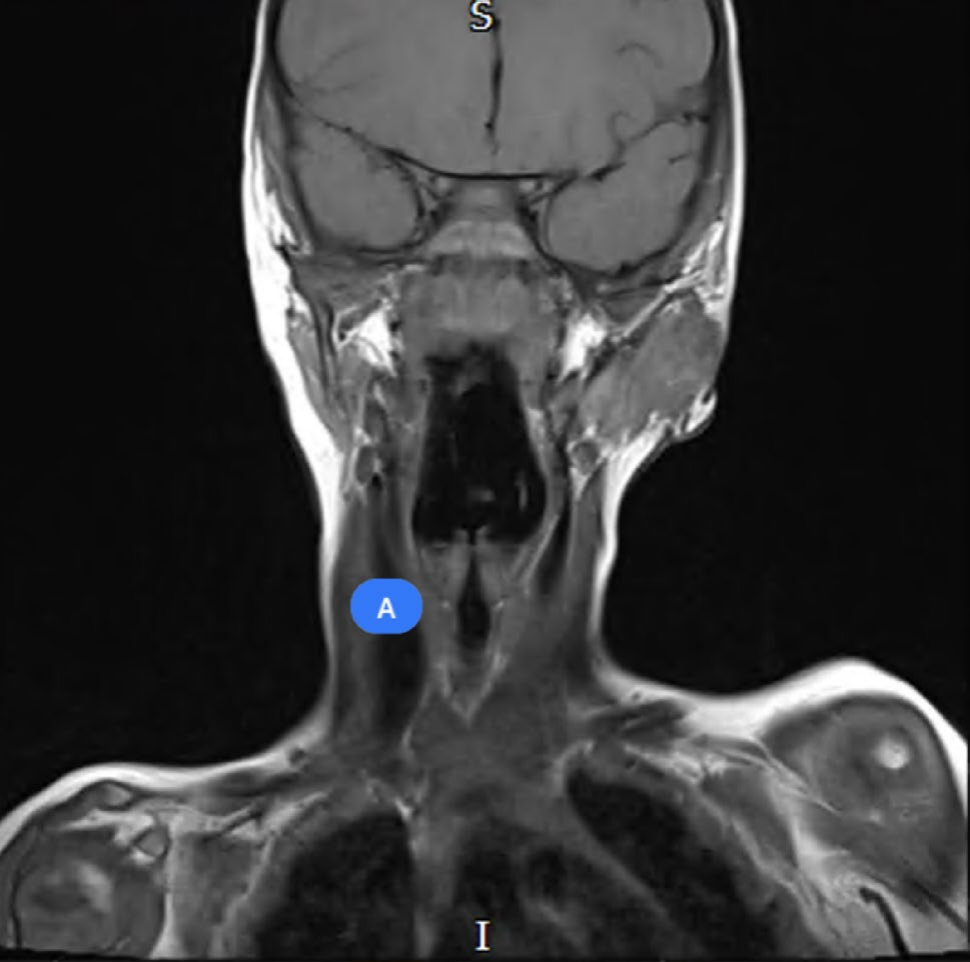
Pre-operative MRI imaging of left first branchial arch cleft cyst
